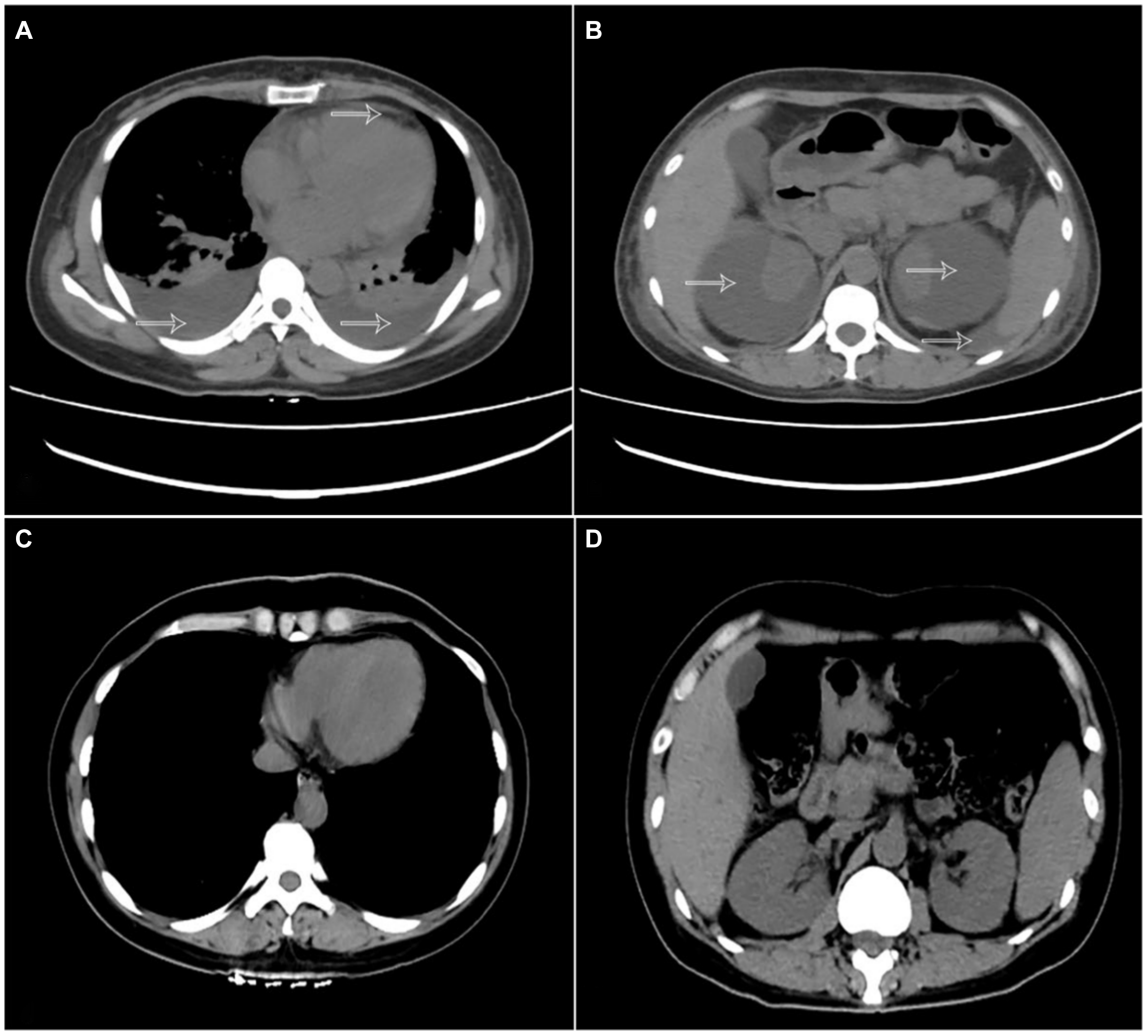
(A) Chest CT showing bilateral pleural effusion and pericardial effusion (arrow). (B) abdominal CT shows subcapsular hematoma of both kidneys and abdominal fluid (arrow). (C,D) CT examination of the chest and abdomen 1 week after surgery indicates pericardial effusion, bilateral pleural effusion, subcapsular hematoma of both kidneys, and abdominal effusion disappeared.
